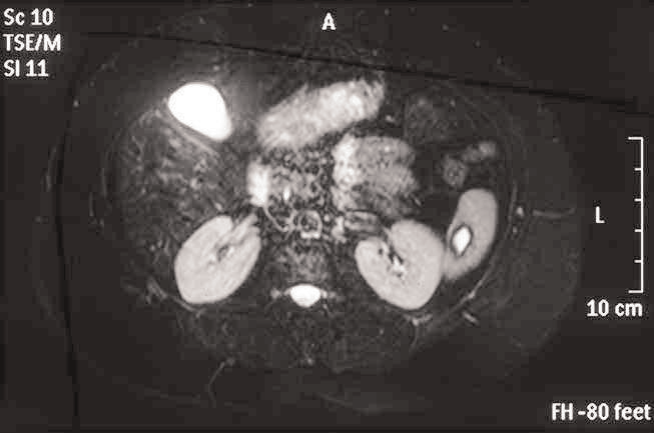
MRI (2006): A 1.8 × 1.4 cm cystic lesion has remained with a low T2 sign in the peripheral border.
